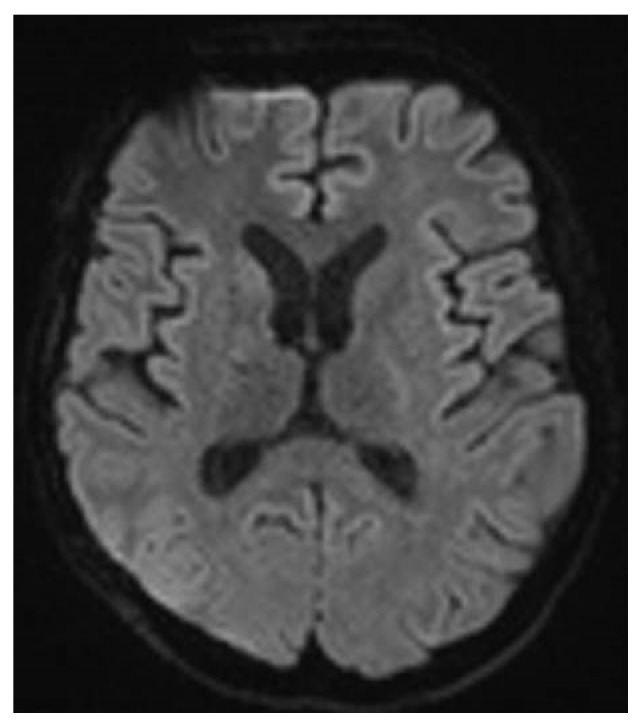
Magnetic resonance imaging at 3 months on DWI sequences showing a regression of punctate hypersignal lesions.
